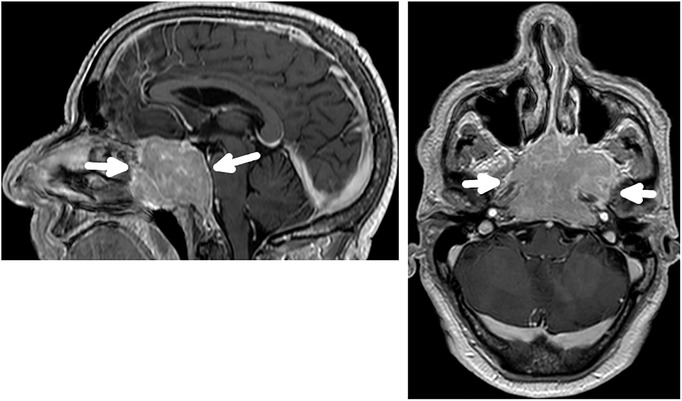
T2‐weighted brain MRI shows an infiltrative lesion centered on the clivus with contrast enhancement invading both cavernous sinuses on sagittal and axial views
